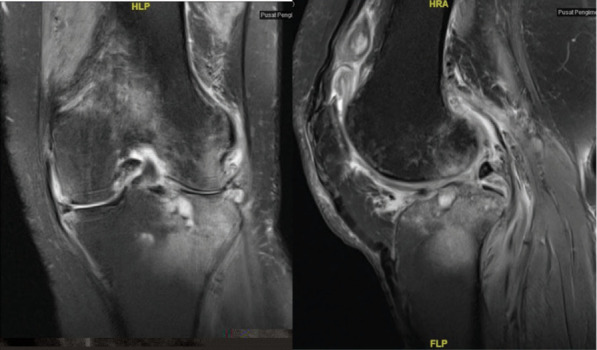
MRI (coronal and sagital) showing osteomyelitis of the medial tibial and femoral condyles.
